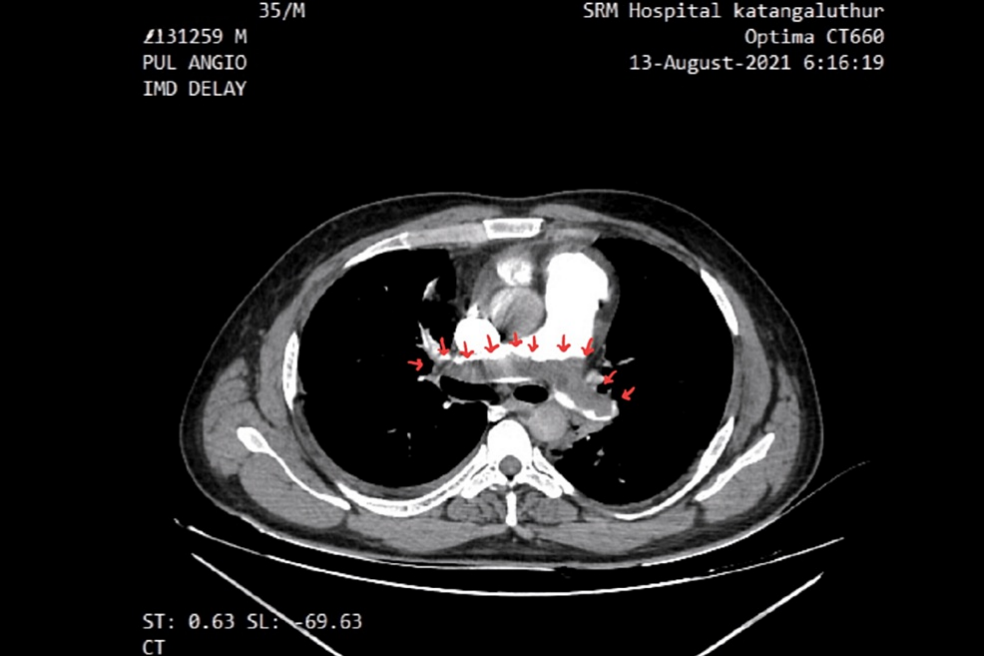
CT pulmonary angiogram showing a large intraluminal filling defect in the main pulmonary artery extending into the right and left pulmonary arteries causing near complete occlusion of the lumen (red arrows)
